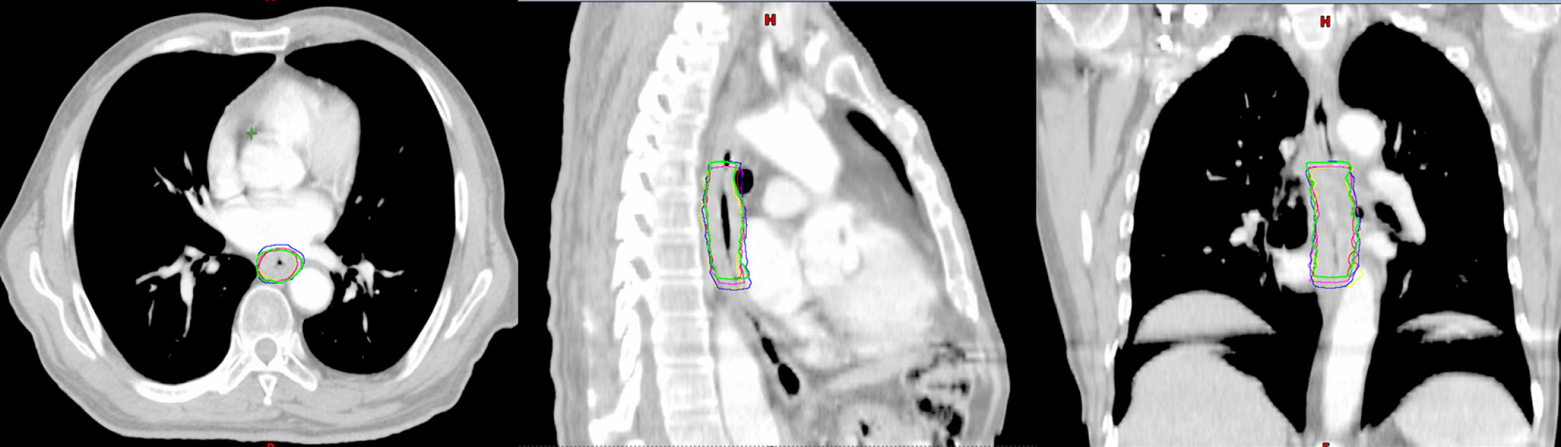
Gross target volume (GTV) or internal GTV (IGTV) formation in one patient: (green), GTV3D; (red), GTV_4D50_; (purple) IGTV_MIP_; (blue) IGTV_10_; (yellow) IGTV_CBCT_ The figures are the axial, sagittal and coronal view of one patient exhibiting the GTV or IGTVs.
